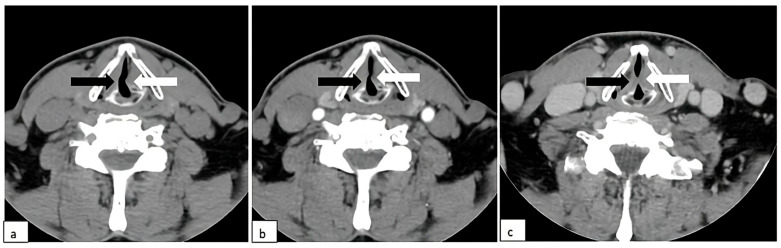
Neck CT: (a) unenhanced scan; (b) contrast-enhanced scan; (c) phonation. Paralyzed left vocal cord (white arrow), fixed and constantly in an adducted paramedian position. Normal appearance of the right vocal cord with adduction in phonation (black arrows) (c).
